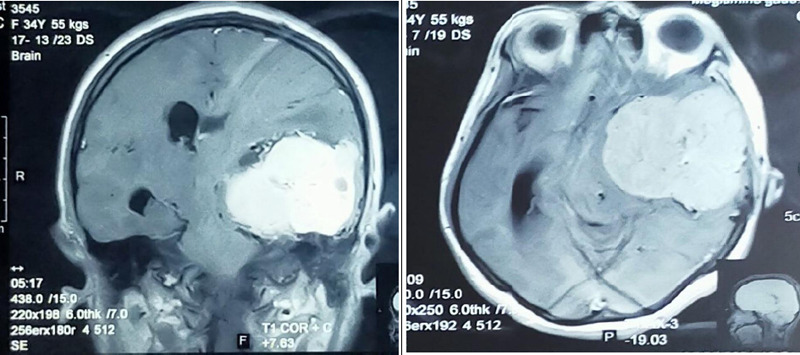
Contrast-enhanced MRI of the brain showing the left-sided hemangiopericytoma with mass effects.
